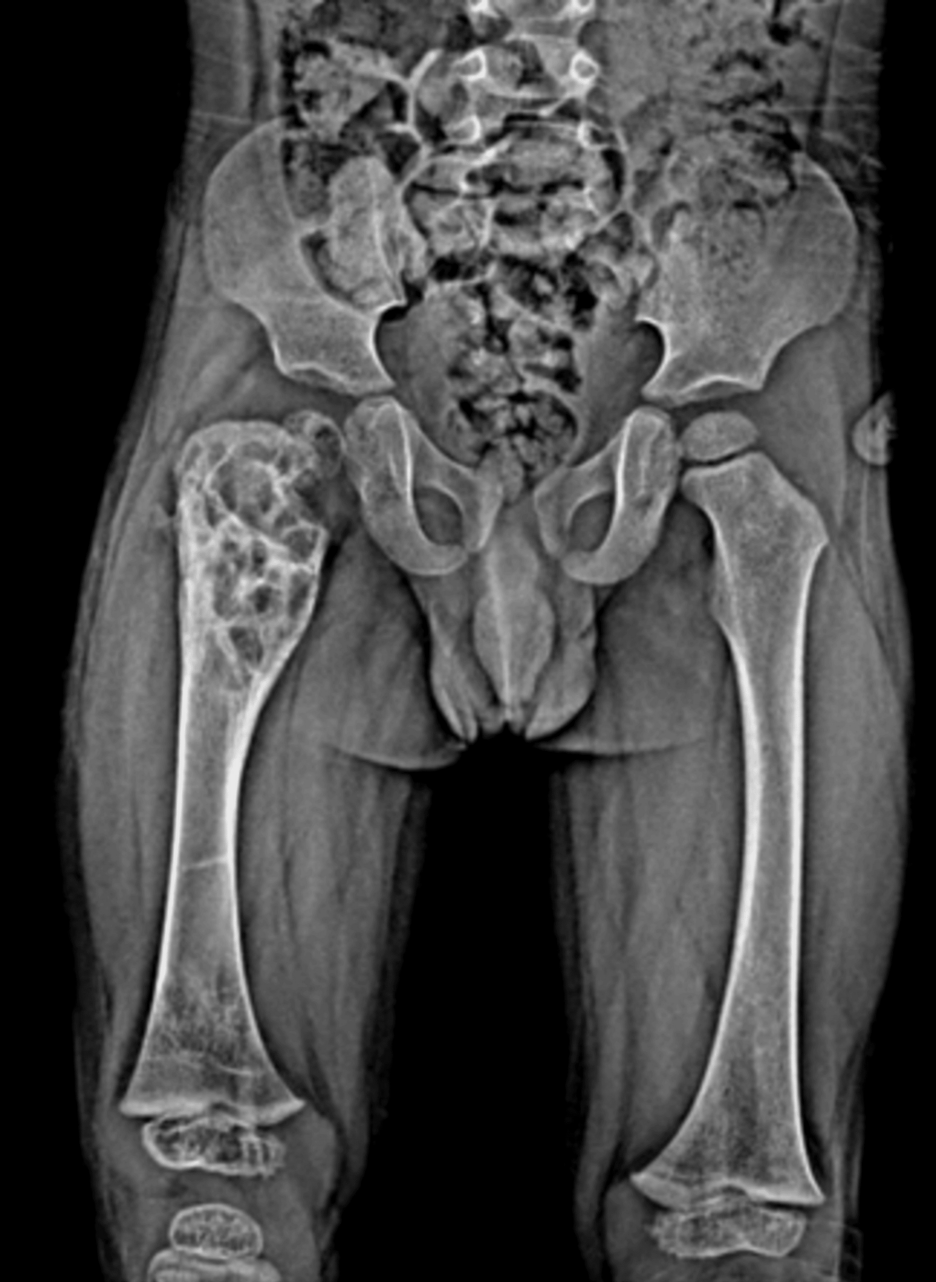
Pathologic fracture in the proximal metaphysis of the right femur on the anteroposterior radiograph of the pelvis
